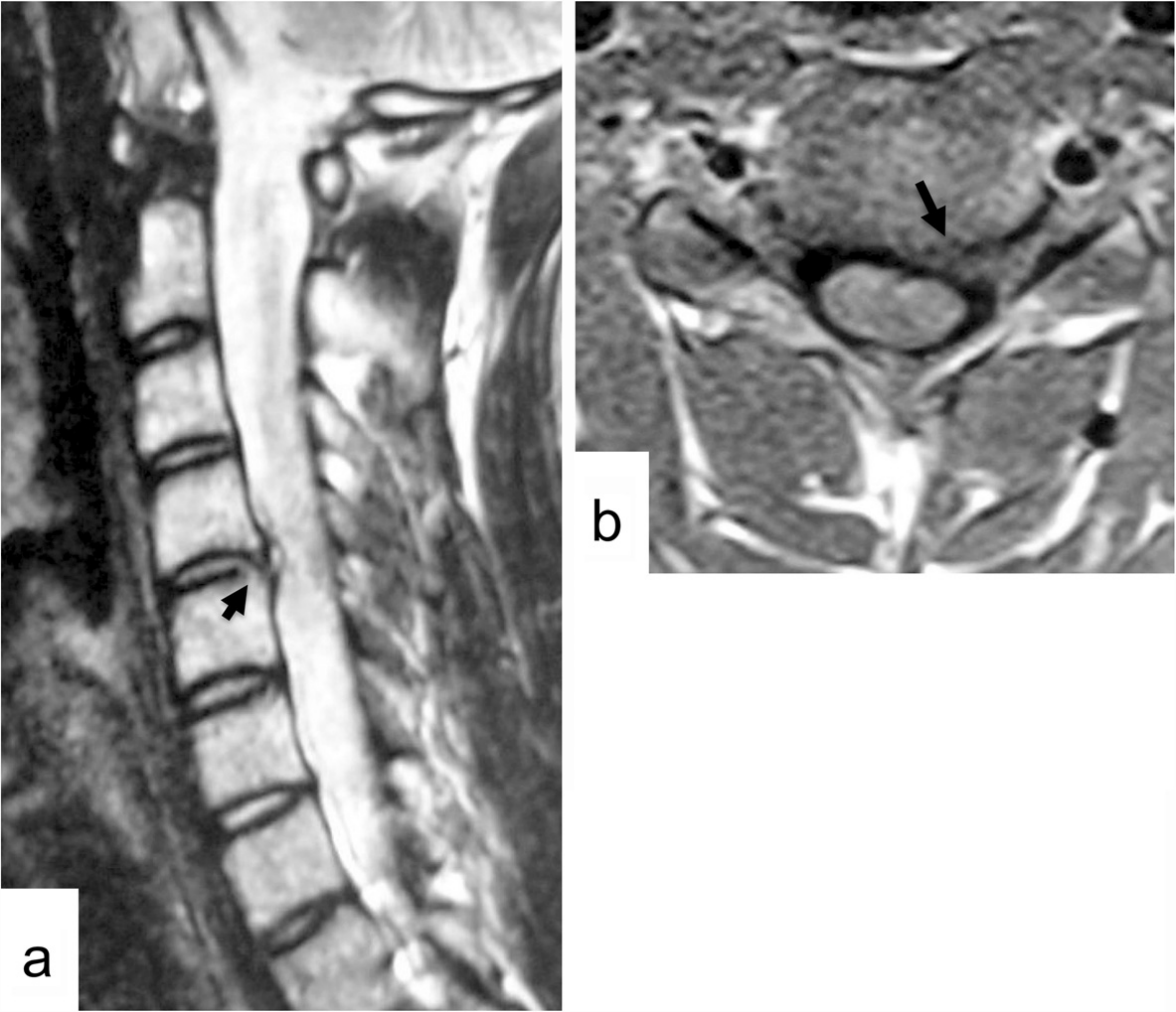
Case 1. Left parasagittal T2-weighted magnetic resonance imaging (MRI) scan of the cervical spine (a) and axial T1-weighted MRI scan at the C4-C5 level (b) showing a herniated intervertebral disc in the left C4-C5 intervertebral foramen (arrows).
